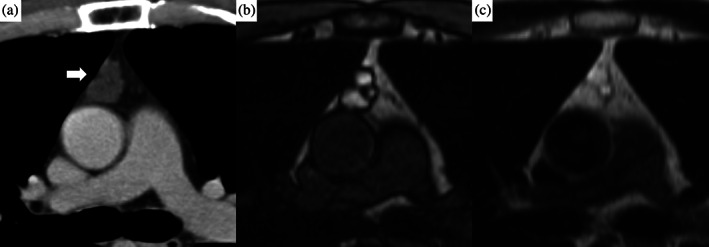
Anterior mediastinal images obtained during the initial examination. (a) Computed tomography imaging with a contrast agent. A 23‐mm, well‐defined, lobular mass was detected (white arrow). (b, c) High signal intensity was detected during T1‐ and T2‐weighted imaging. Because a multilocular wall was detected, the mass was considered to be a multilocular thymic cyst.
